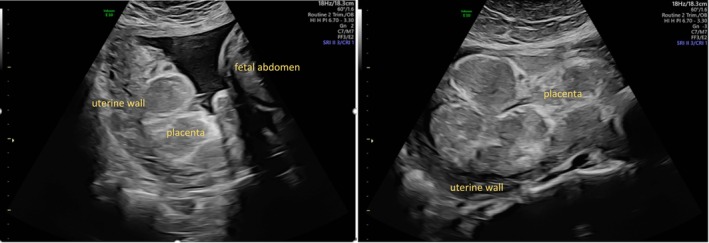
Ultrasound placenta case 2 (31 + 6 weeks of gestation). Significant calcifications appear early on in pregnancy.
